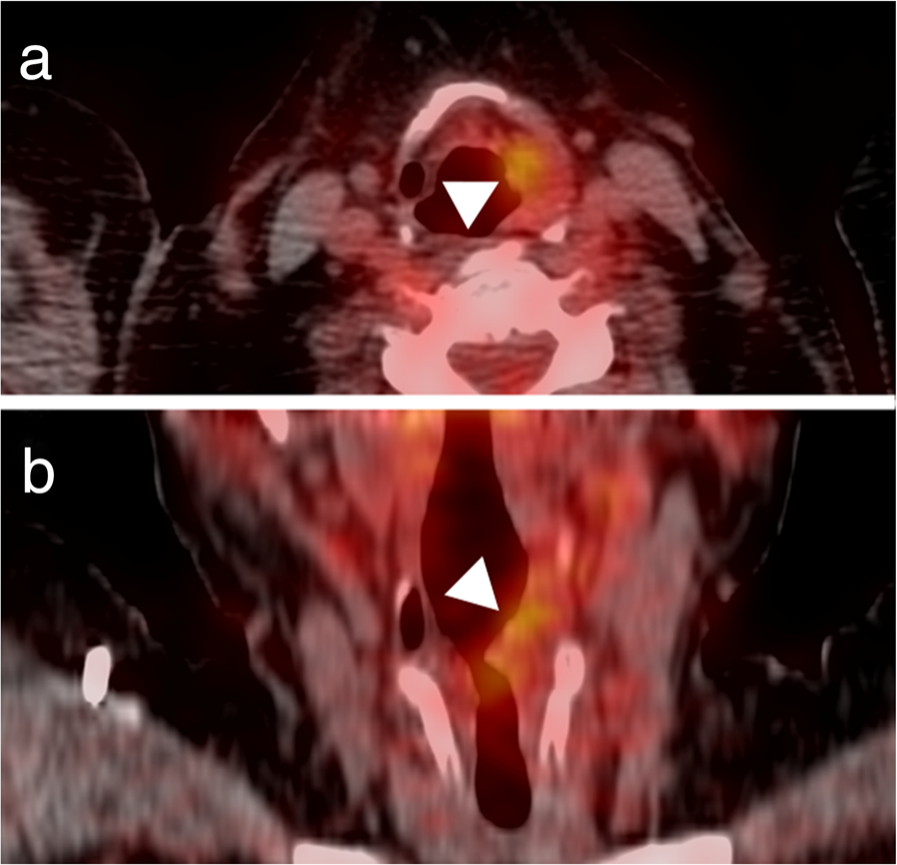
18F-FDG-PET/CT of the larynx. Intense FDG uptake in the left supraglottic region (arrowhead). a) axial, b) coronal
